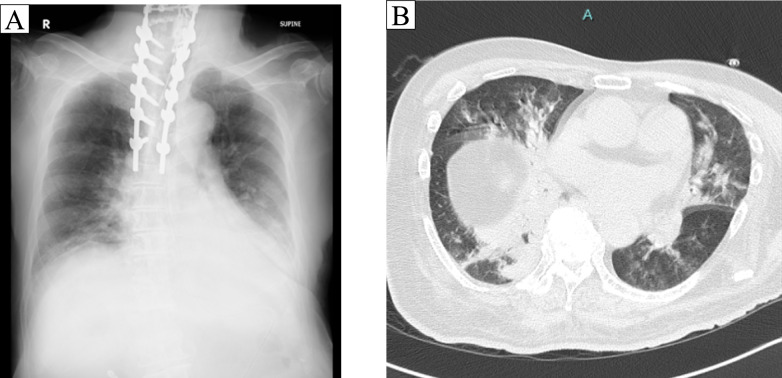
The chest radiograph and chest computed tomography. Both chest radiograph and chest CT revealed consolidation in the right lower lobe
